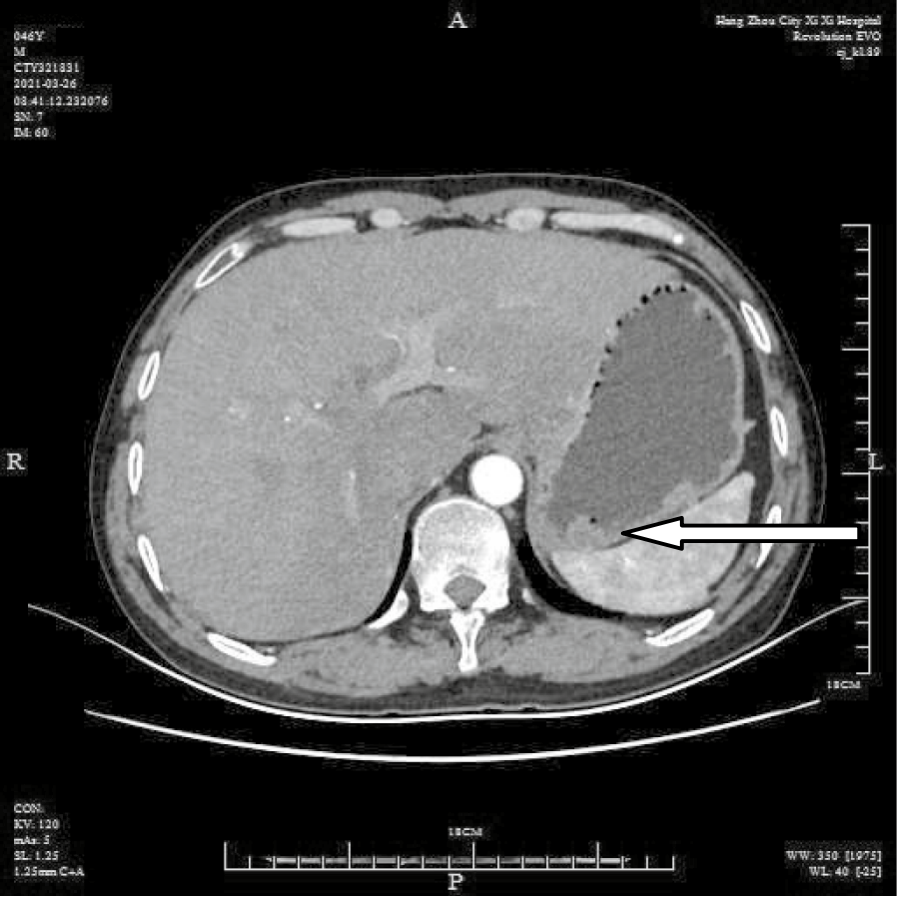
Slightly thickened stomach wall in CT after one month treatment
